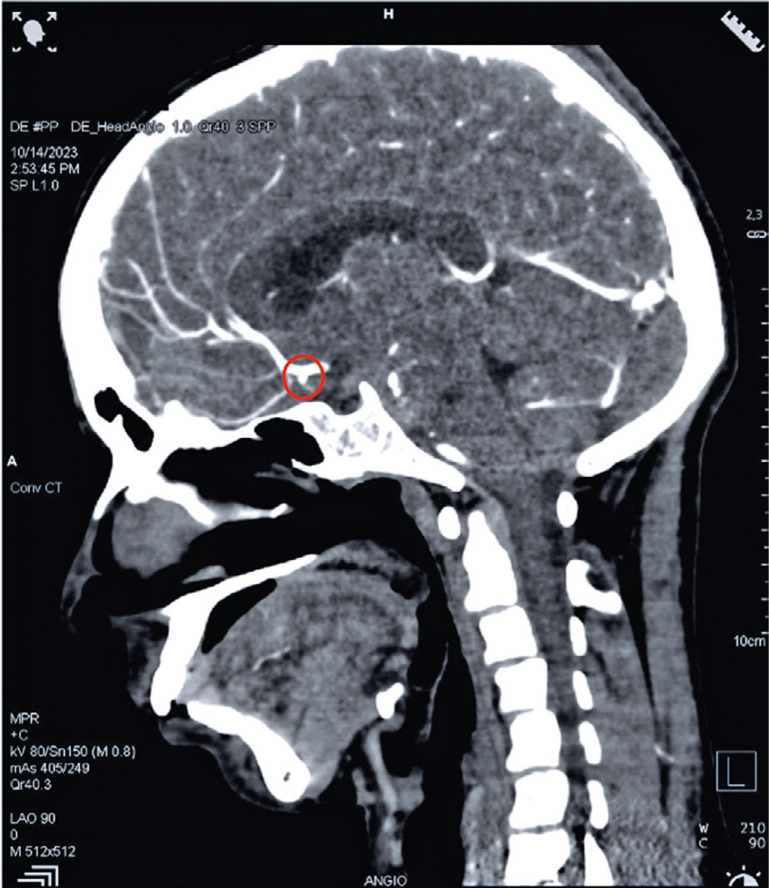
Head CT (sagittal view) of an outpouching left of the ACA-ACom junction (red circle)
